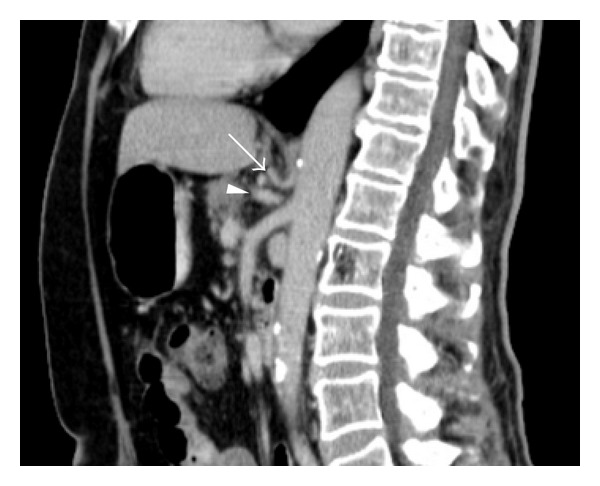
Sagittal CT scan image demonstrating the supra-diaphragmatic origin of the left gastric artery (arrow) and the separate celiac origin (arrowhead). Aortic atherosclerotic calcifications can also be seen.
